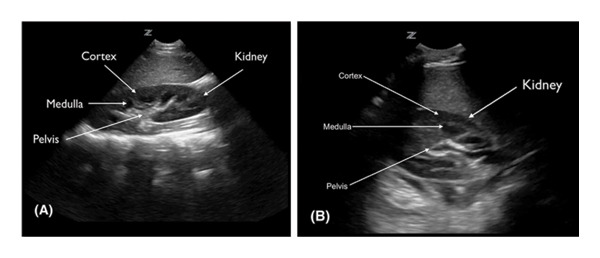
Normal Sonographic Appearance of a Pediatric Patient’s Right Kidney at (A) longitudinal and (B) transverse plane.
